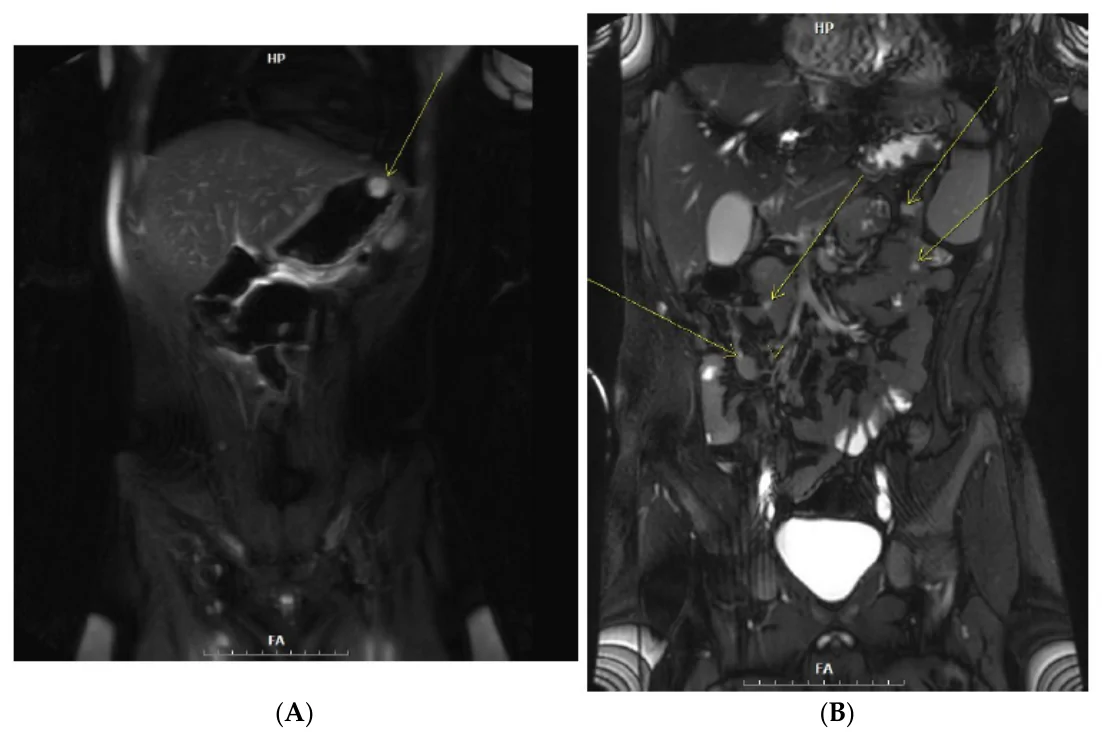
Coronal T2-weighted MR enterography. (A) A small hyperintense polypoid lesion in the gastric fundus (arrow), consistent with a venous malformation. (B) Additional hyperintense small bowel lesions of varying size (arrows), indicating involvement not fully captured by endoscopy.
